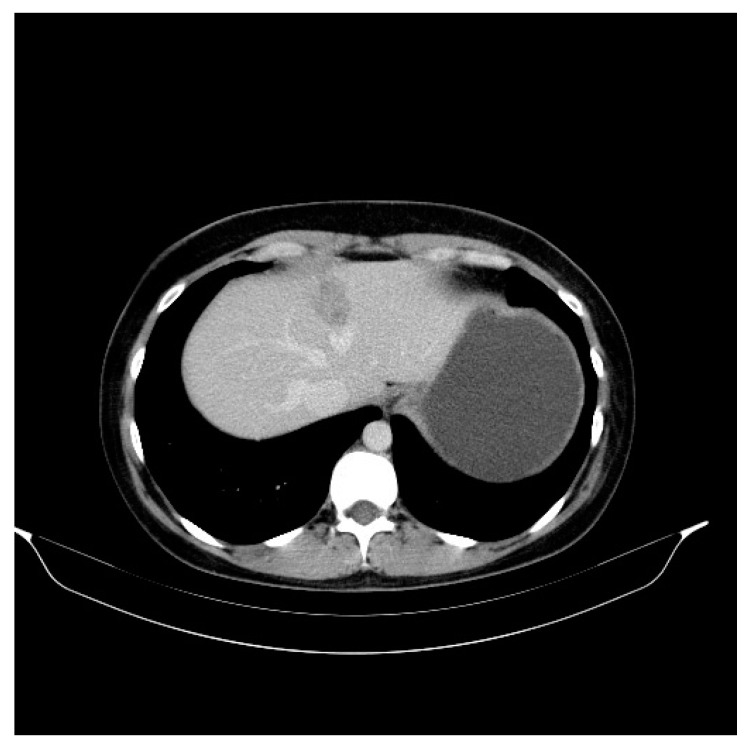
Liver metastasis in 31-year-old women with infiltrative ductal breast carcinoma (IDC), G2, with an “in situ” ductal component of the comedo type. Axial noncontrast abdominal CT image performed in 2016 shows a liver metastasis (31 mm diameter) with an ill-defined area of low attenuation and faint high attenuation.
